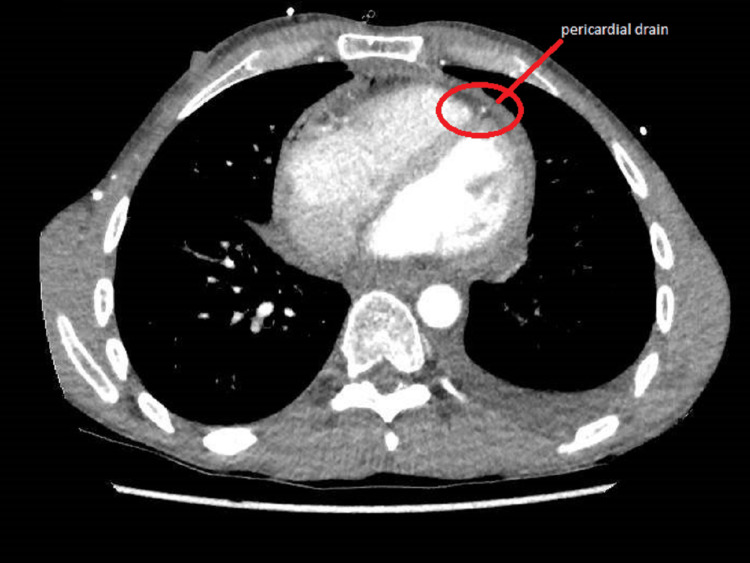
CT of the chest, abdomen, and pelvis Minimal residual pericardial fluid with the pericardial drain in situ.
